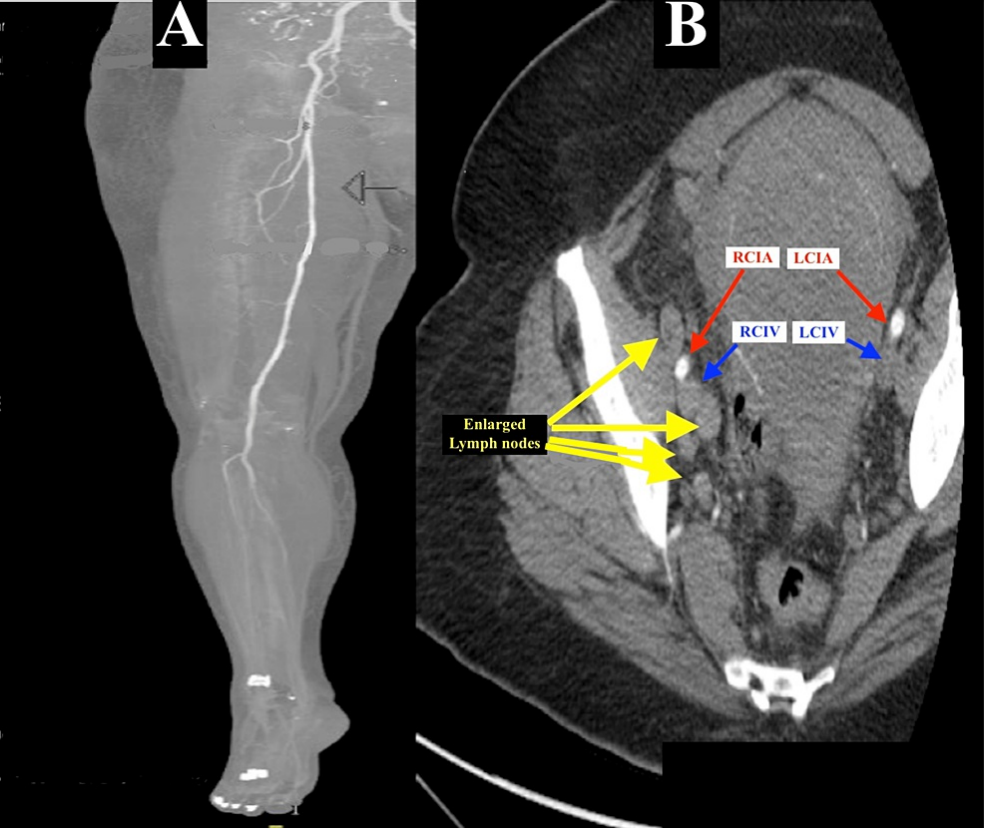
Computed tomography angiography of the right lower extremity and pelvis (A-B): Enlarged iliac chain lymphadenopathy worse on the right than left (yellow arrows), along the right pelvic sidewall abutting the right common iliac artery (red arrow) and compressing an enlarged iliac vein (blue arrow), effacing the right external iliac vein and likely causing the iliotibial DVT distally (not shown). Notice the significant edema and swelling of the right thigh and leg (A) with no arterial occlusive disease (grey arrow). DVT: deep vein thrombosis; RCIA: right common iliac artery; LCIA: left common iliac artery; RCIV: right common iliac vein; LCIV: left common iliac vein.
